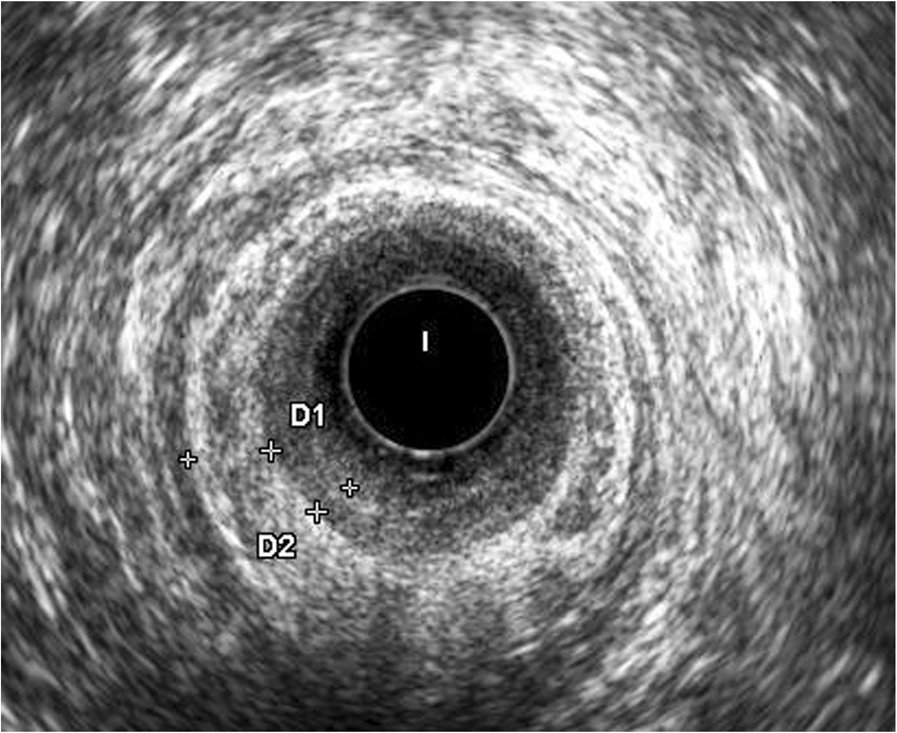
Endoanal ultrasound (US) after treatment. It is evident a complete regression of the anal tumor.
